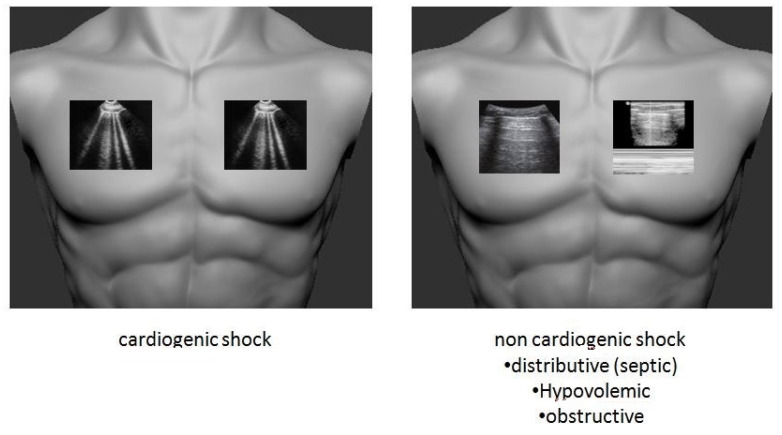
Lung sonography protocol for undifferentiated shock.
